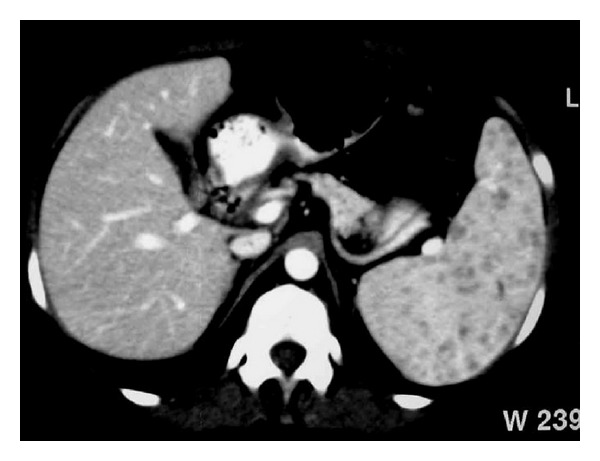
NHL. Axial contrast-enhanced CT image demonstrates multiple low-attenuation nodules in the spleen.
